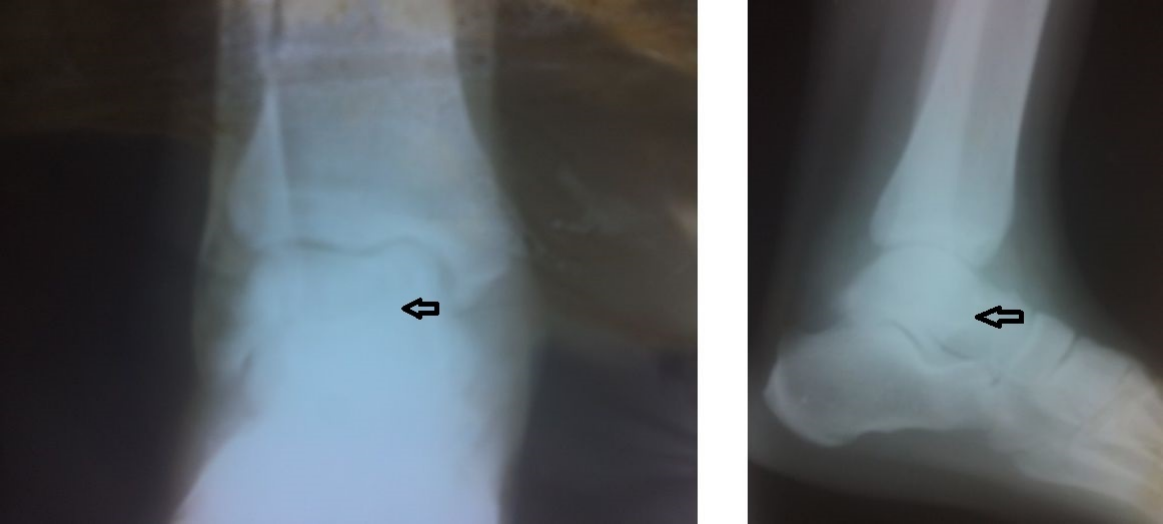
Follow-up plain radiographs of the right ankle's anteroposterior and lateral views. Healed lesion in the talus (black arrows) after 12 months of anti-tubercular chemotherapy.
